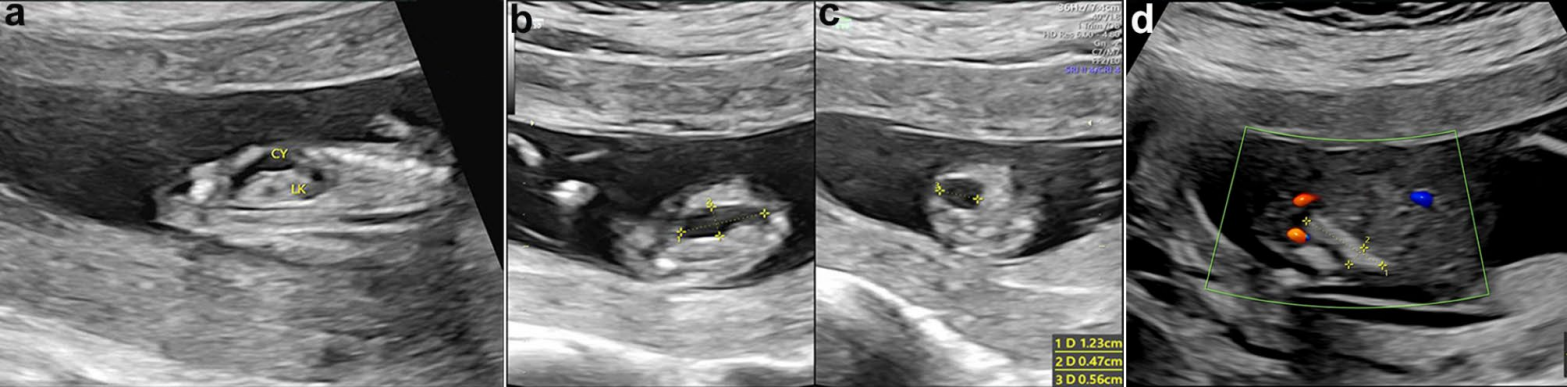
Ultrasound (US) images show the evolution of fetal abdominal cyst (CY) from 12 and 15 weeks of gestation, respectively. (a) The sagittal US image of the fetal abdomen depicts a bowel-like cystic structure lying anterior to the left kidney (LK) at 12 weeks of gestation. Transverse (b) and longitudinal (c) views of the cystic structures measuring 12 × 6 × 6 mm (cursors). (d) Follow-up ultrasound examination at 15 weeks of gestation shows the echogenic bowel in the left lower abdomen measuring 16 × 4 mm (cursors); the cyst was not visible
